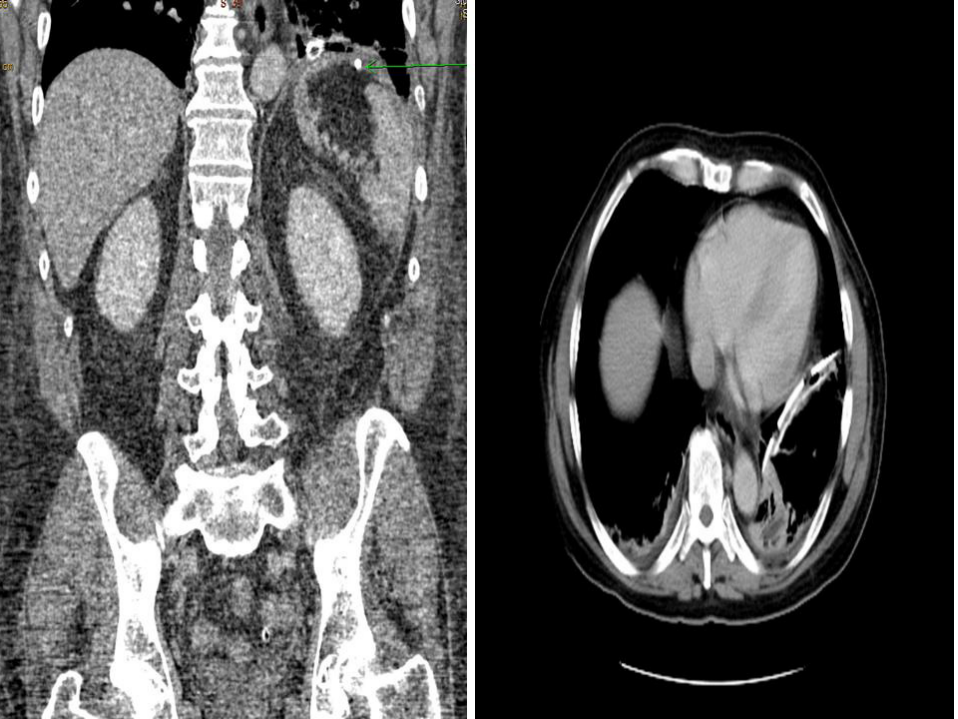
(a and b): Post operative CT (Coronal and axial views). Note the repaired left diaphragam and tip of the chest drain in situ with some patchy basal consolidation (Arrow pointing to protec stapler).
